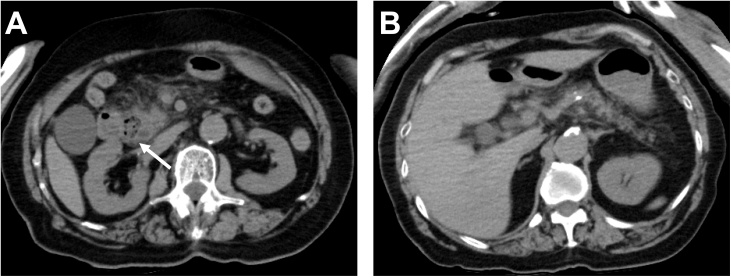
Plain axial CT of the abdomen and pelvis. Plain axial CT of the abdomen and pelvis demonstrated (A) inflammatory changes around the pancreatic head and large periampullary diverticulum (arrowhead). (B) A coronal slice showing no inflammatory changes around the pancreatic body and tail.
